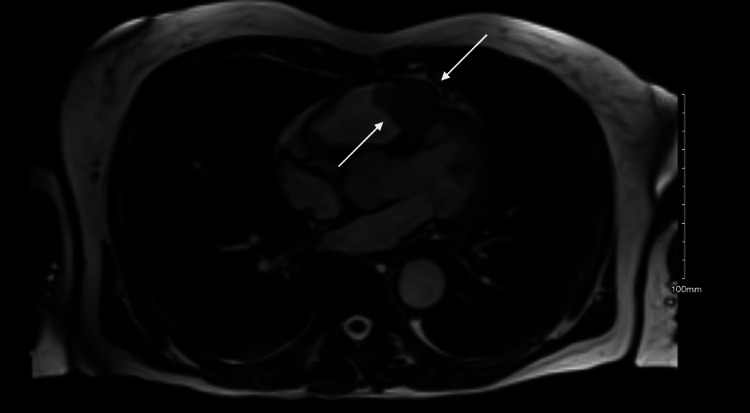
Cardiac MRI Axial view from cardiac MRI showing RV with mass identified with white arrows. RV: right ventricle
